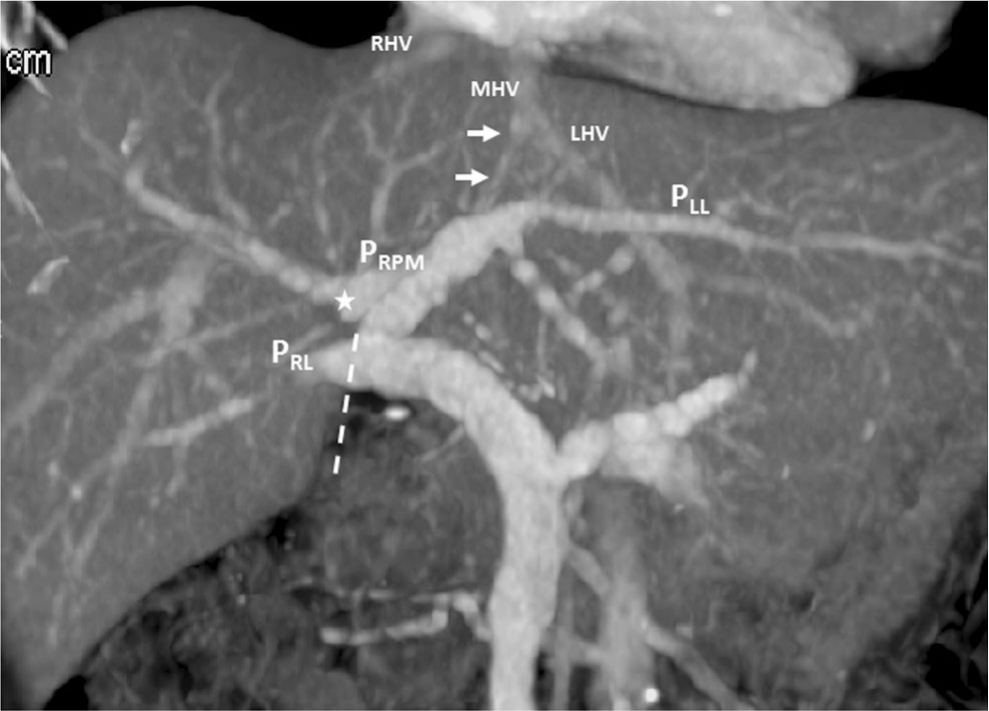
Contrast-enhanced CT with MIP reconstruction revealing portal vein ramification of a Shindoh’s independent right lateral type, with the umbilical portion (*asterisk*) of the portal vein tilting to the right and joining with the RSLT (*dotted line*) and the MHV running to the left (*arrows*) of the RSLT
